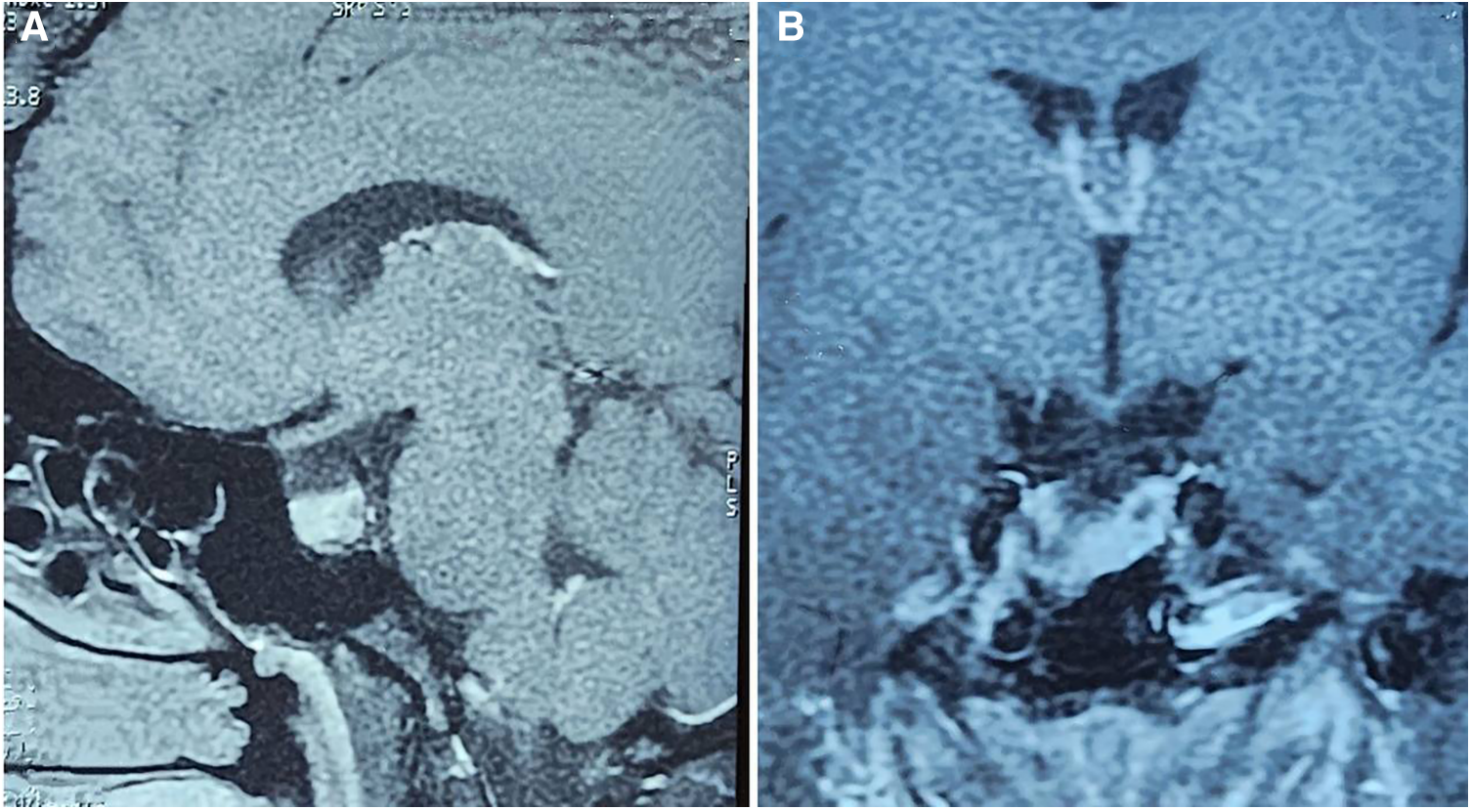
Sagittal (A) and coronal (B) views of dynamic MRI of the sellar region in patients with empty sella.
